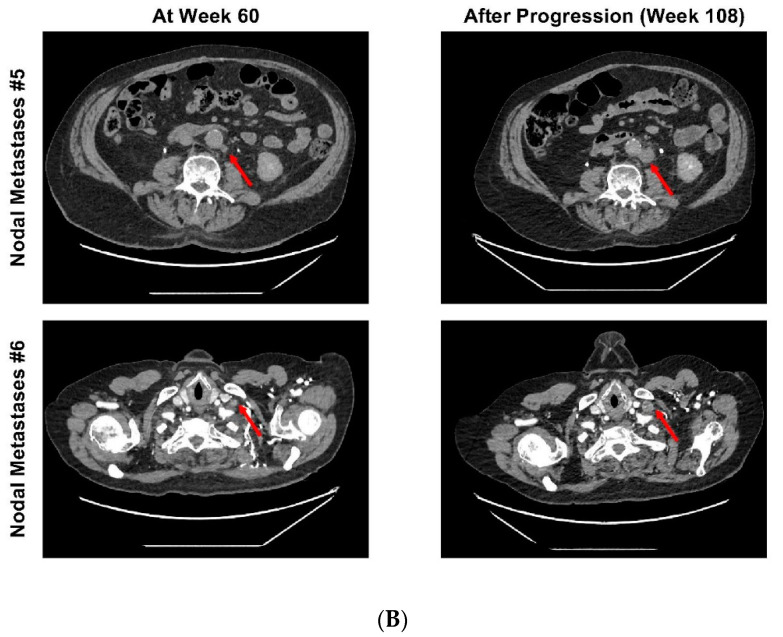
(A) Initial tumor scans (at start of rucaparib treatment) compared with scans at the time of best response (week 60 of rucaparib treatment) of nodal metastases and lytic bone metastases at the target lesions. (B) Scans at the time of best response (week 60 of rucaparib treatment) compared with scans after radiographic progression.
